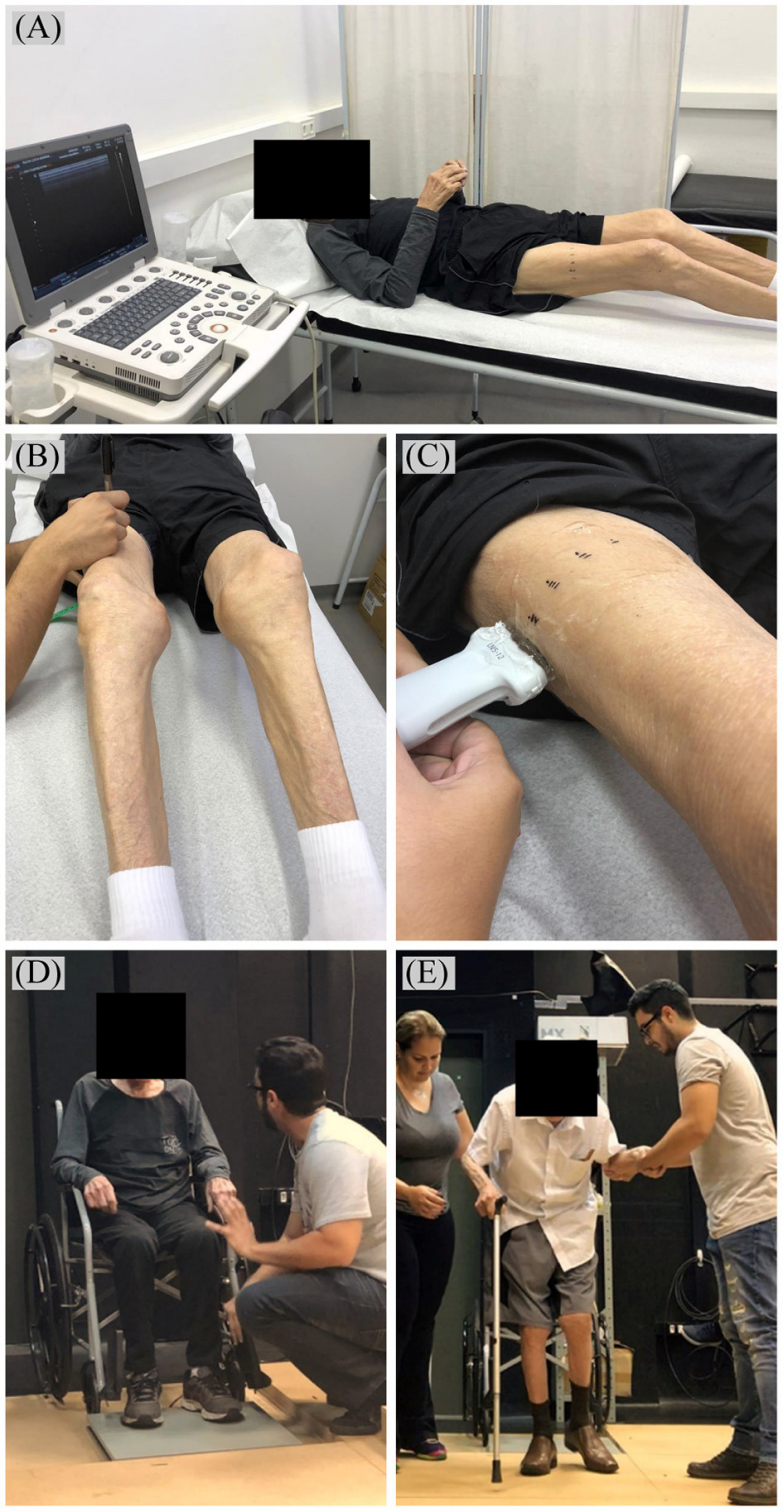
(A) Positioning and (B) skin markings for ultrasound image acquisition of vastus lateralis cross-sectional area and (C) muscle thickness (with ultrasound probe oriented longitudinally to the muscular belly). Timed Up and Go Test (D) instructions and (E) execution.
